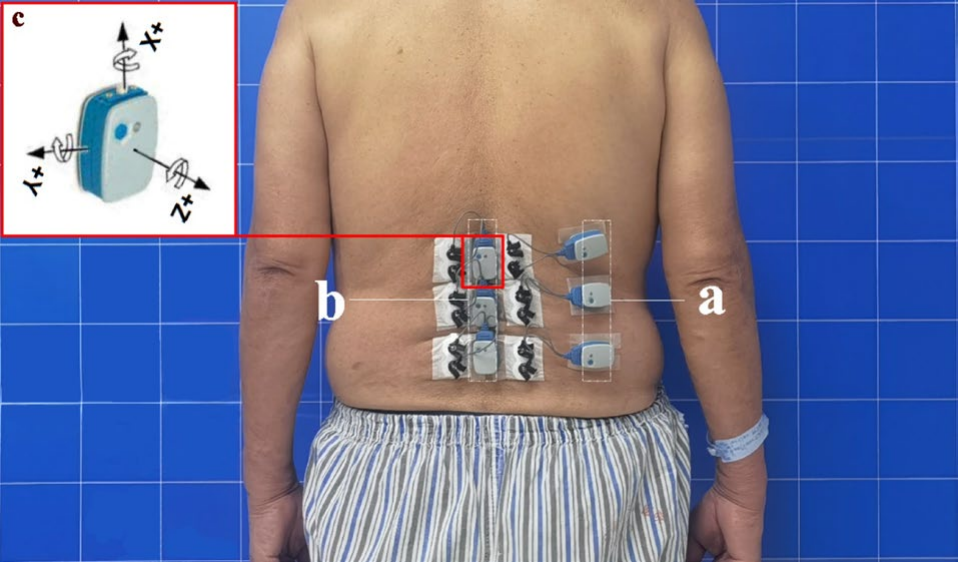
Six bipolar electrodes were placed at three levels of the bilateral PVMs (UEV, AV and LEV). After confirmation by radiographs, the sEMG sensors and IMUs were placed in the appropriate areas. (a) Three sEMG sensors on the right. (b) Three sEMG sensors on the left, each integrated with an IMU, placed on the corresponding position of the spinous process (L1, L3 and L5). (c) Three axes of the IMU.
